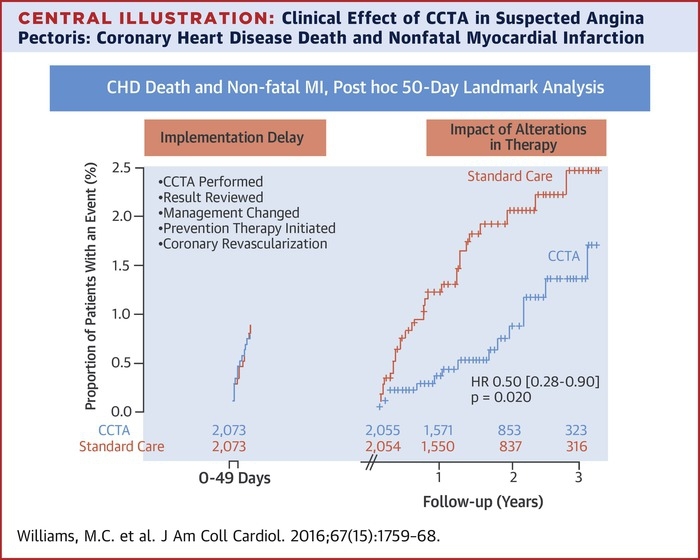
Clinical Effect of CCTA in Suspected Angina Pectoris: Coronary Heart Disease Death and Nonfatal Myocardial Infarction Post hoc landmark analysis at 50 days to account for the implementation and treatment delay consequent on the conduct, reporting, and communication of the coronary computed tomography angiography (CCTA) findings. HR = hazard ratio.
